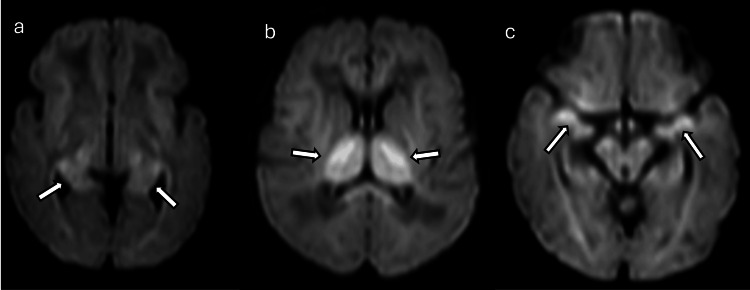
A four-day-old patient with hypoxic ischemic encephalopathy (a), (b), and (c): Axial DWI sequences demonstrate central/basal ganglia–thalamus pattern injury with symmetric restricted diffusion involving both thalami, splenium, posterior limb of internal capsule, corticospinal tract, and hippocampi (arrows). Images obtained by the authors from the institutional PACS. All images are de-identified and used in accordance with institutional policy. DWI: diffusion-weighted imaging; PACS: picture archiving and communication system
